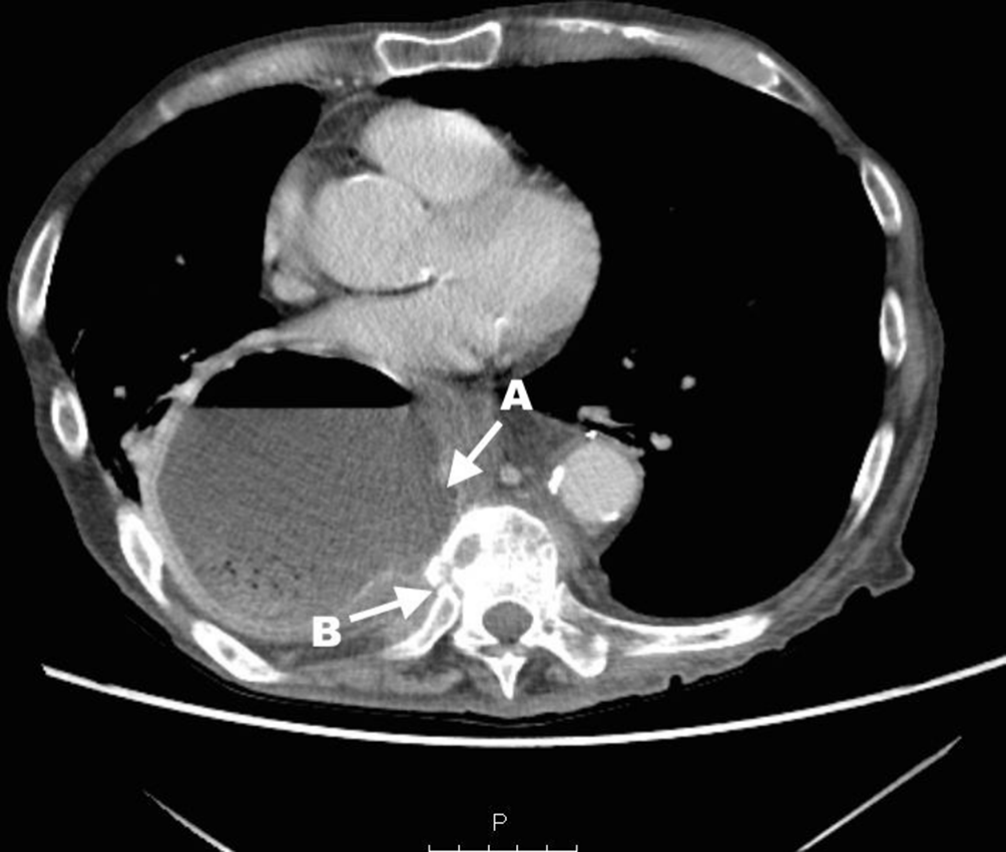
Axial CT image showing a diverticulum arising from the postero medial wall of the stomach (arrow A) which is sited in the mediastinum. The resulting destructive process of the T8 vertebral body can also be seen (arrow B).
